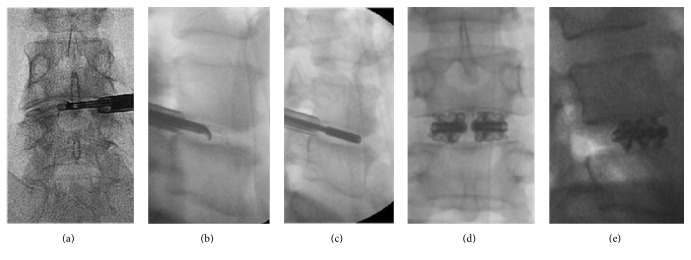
A 37-year-old male patient (patient number: 15 in the tables) with DDD and HNP at the L4-5 level. Lateral standing X-rays showing (a) preoperative, (b) early postoperative, and (c, d, e) final follow-up X-rays including standing lateral neutral, extension, and flexion views taken at 50 months after the surgery. Note that there is 1.9 mm reduction of the DH at the final follow-up examination compared to the early postoperative period. The operated level remained stable in extension and flexion views. The patient's VAS-B, VAS-L, and ODI scores were 1.4, 2, 4.4, respectively, at the final follow-up visit. The patient rated his result as “excellent.”
